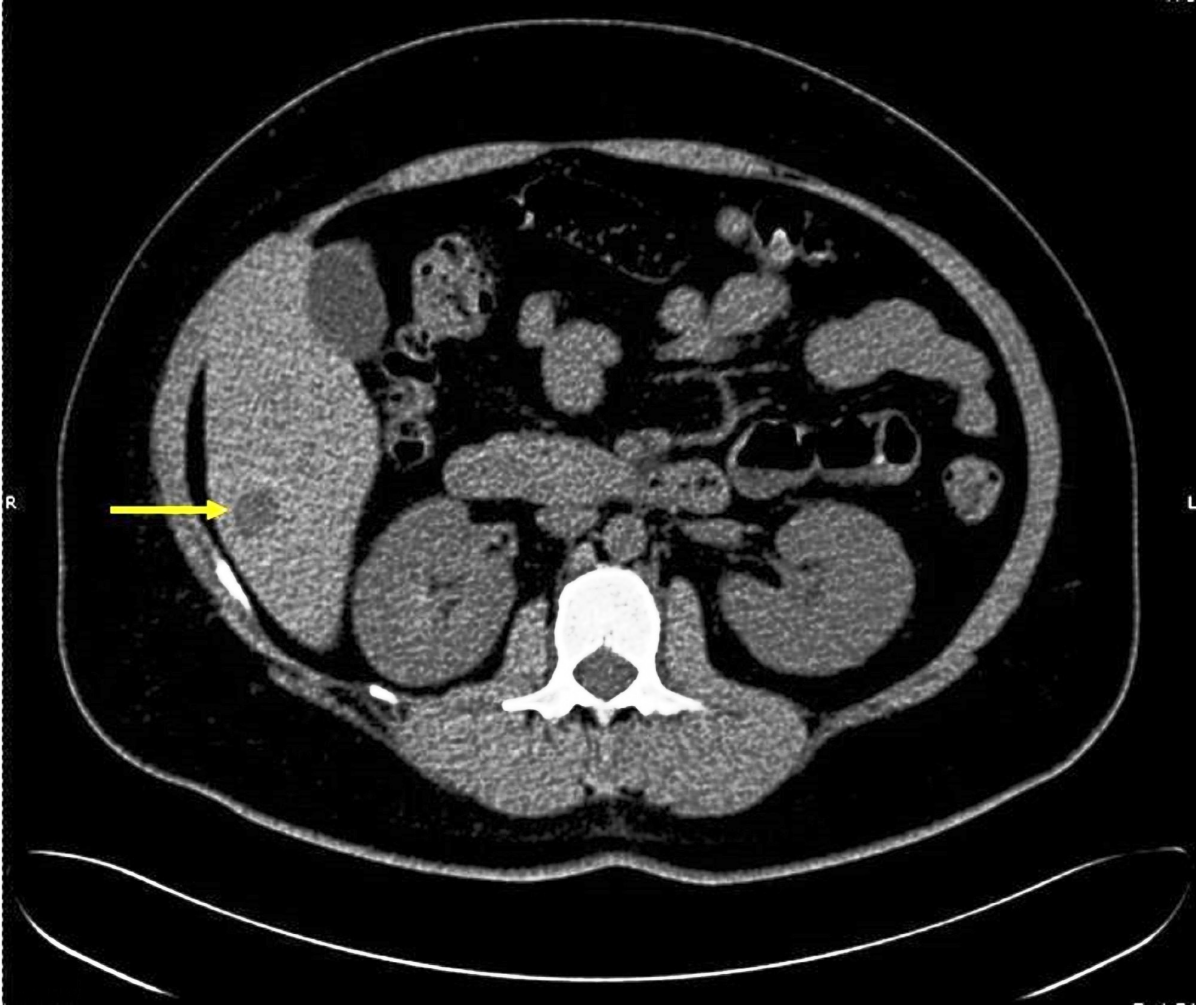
CT of the abdomen A CT scan of the abdomen without contrast showing a 1.6-cm hypodense lesion at the inferior aspect of the right hepatic lobe.
